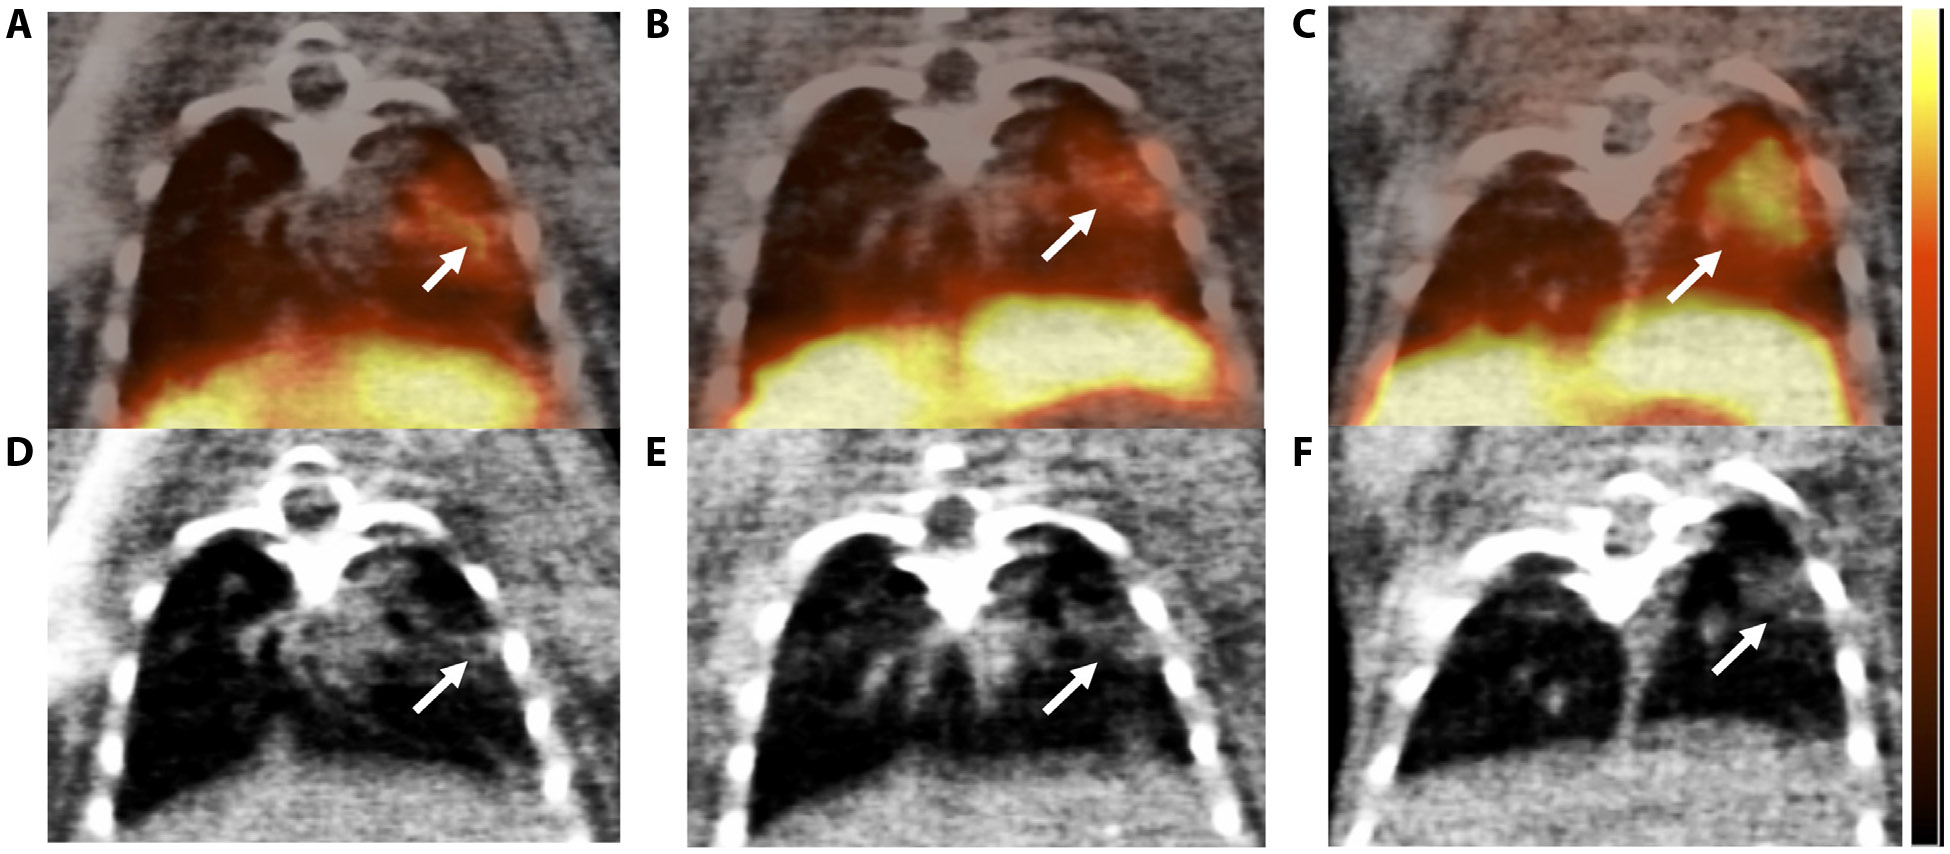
Representative PET/CT images for the focal ablative RIPF model up to 8 weeks post-RT. Images were acquired at 1 hour postinjection of 11.1 MBq of [^64^Cu]Cu-*α*_*v*_*β*_6_-BP at 4 to 8 weeks post-RT (70 Gy delivered focally to a 3 × 3 mm spot in the left lung using an AP:PA configuration). The upper panels (A-C) correspond to PET/CT fusion images (arrows indicate the uptake region), and the lower panels (D-F) show CT images only (arrows indicate the damaged area). Uptake was observed at week 4 (A); the CT image indicated lung tissue damage (D). At week 6, a more diffuse uptake was noticed by PET (B), whereas CT revealed similar lung tissue damage (E). At week 8, there was a more intense and diffuse uptake of [^64^Cu]Cu-*α*_*v*_*β*_6_-BP (C), which was validated by the larger and more diffuse area of lung tissue damage by CT (F). The color bar on the right side represents the SUV mean of 0-0.5. *Abbreviations:* PET/CT = positron emission tomography/computed tomography; RT = radiation therapy; SUV = standard uptake value.
